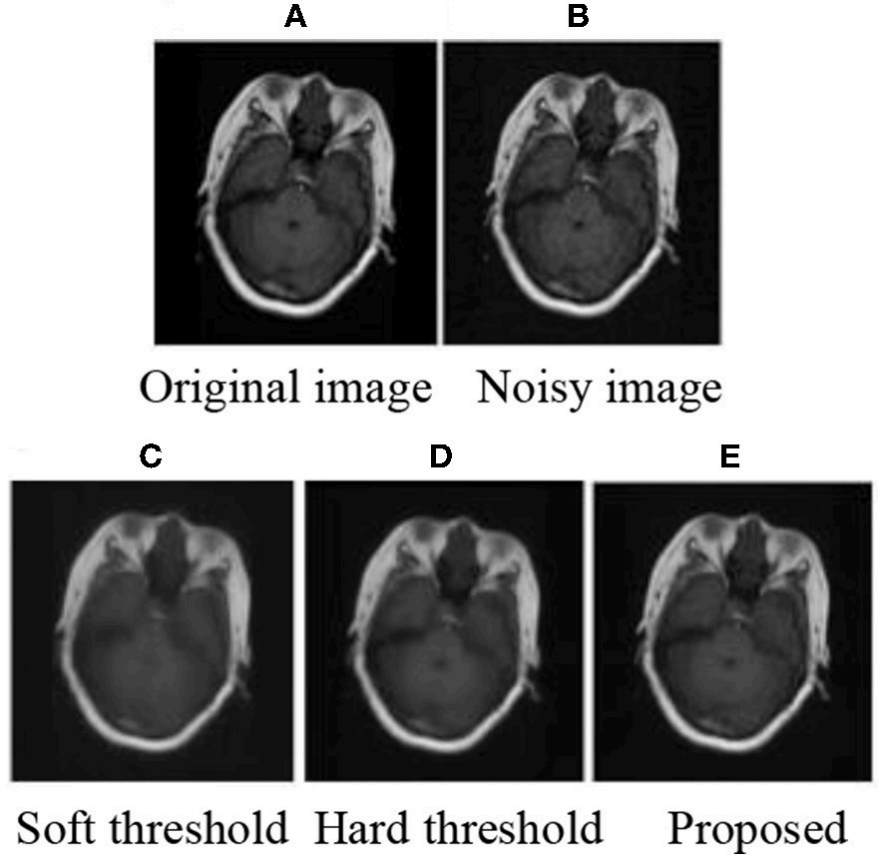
MRI 3 of subjective results.
